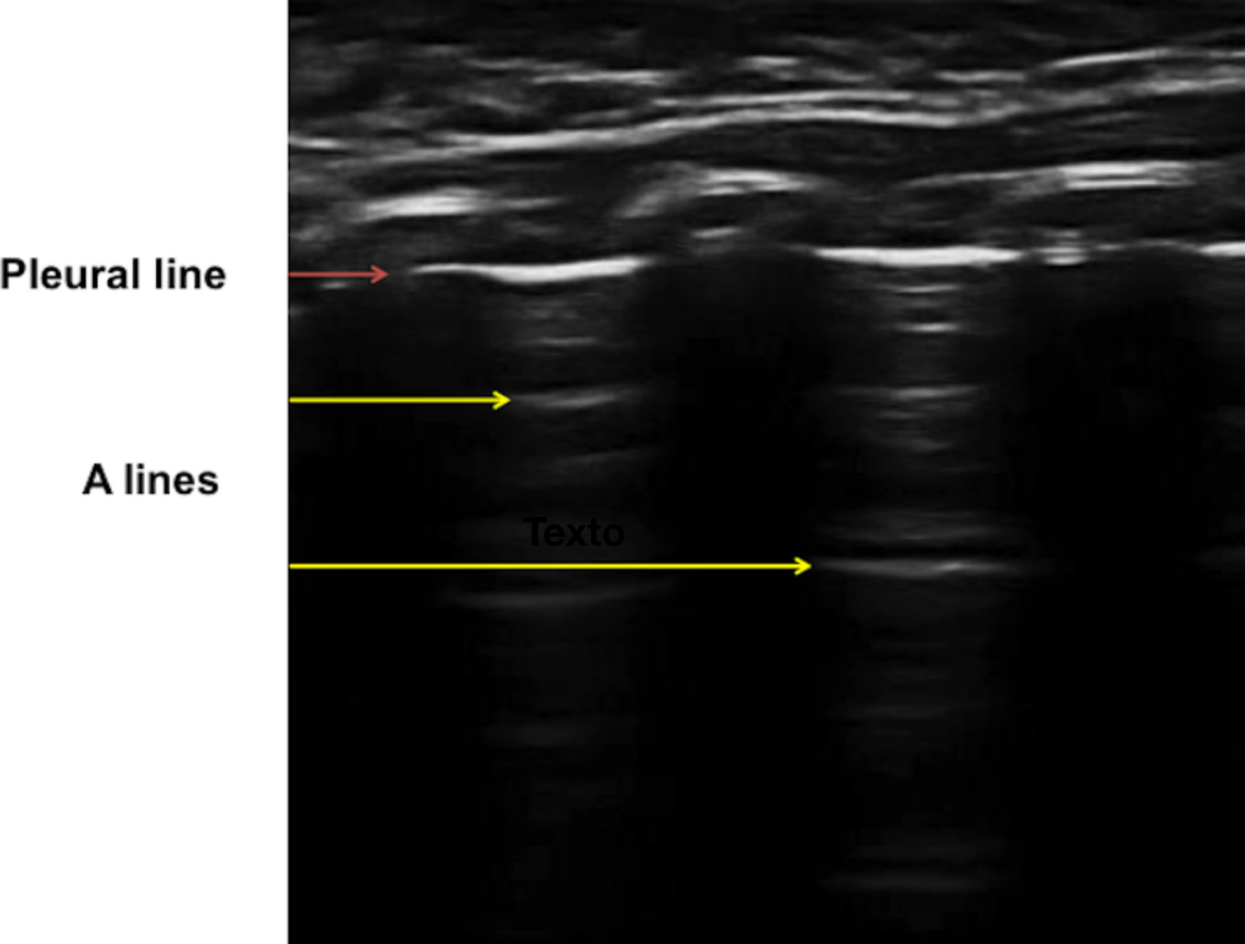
Ultrasound of the lung with the echogenic pleural line and the horizontal artifacts “A lines“.
